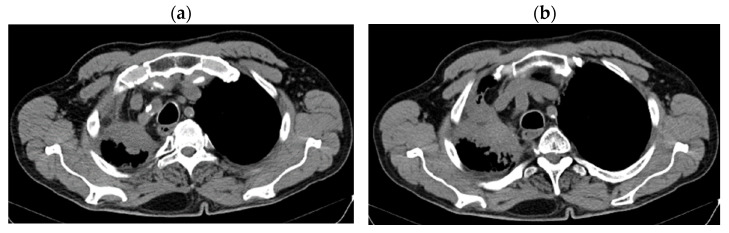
(a,b) CT from 30/03/23 vs. CT from 09/2022: DISEASE PROGRESSION (primary tumor growth by 22.32%: 33 × 51 × 53 vs. 24 × 46 × 40 mm).
